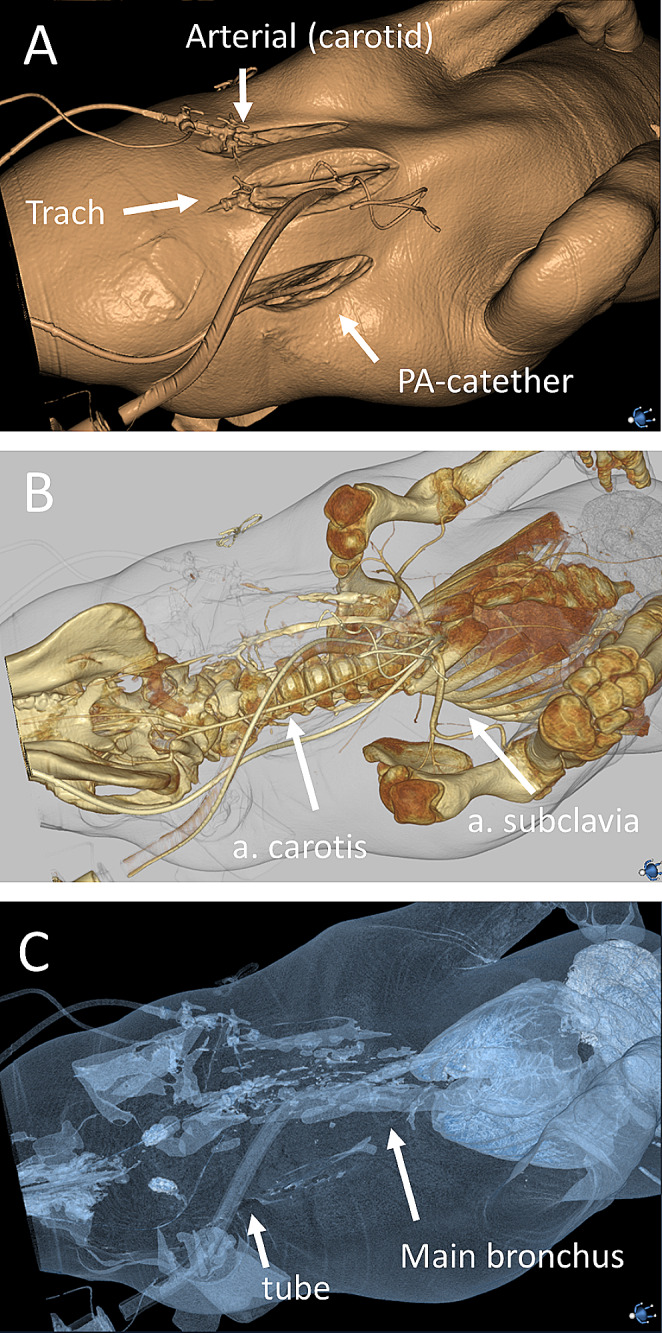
3D renderings of computed tomography showing vascular and ventilatory accesses. (A) The PA-catheter is placed on the right side of the trachea by cut-down technique. Tracheostomy (or intubation) in the trachea. An arterial line may be placed in the left internal carotid, if not placed in the foreleg
